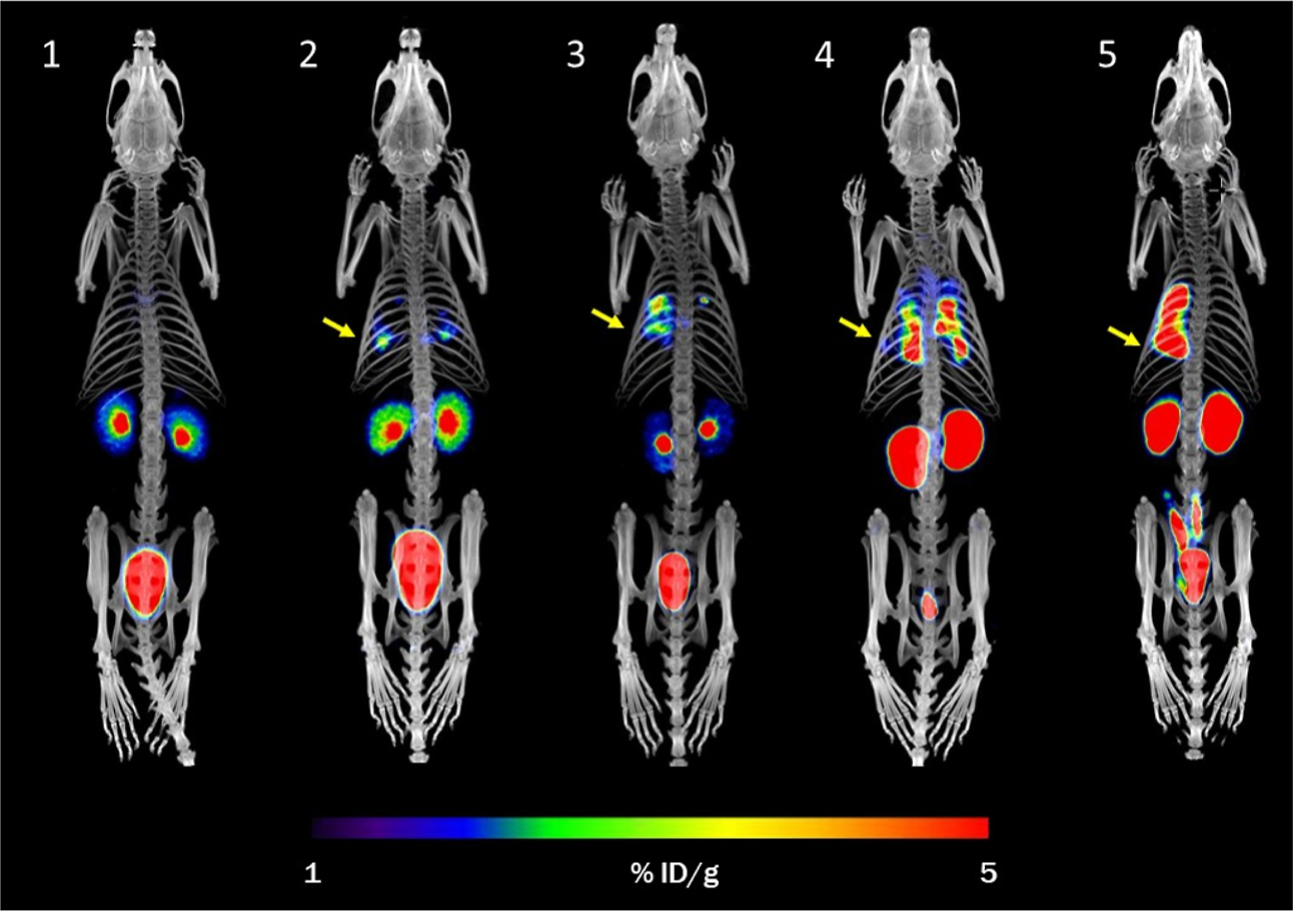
PET/CT MIP images of [^68^Ga ]Ga-ORNB in a control
